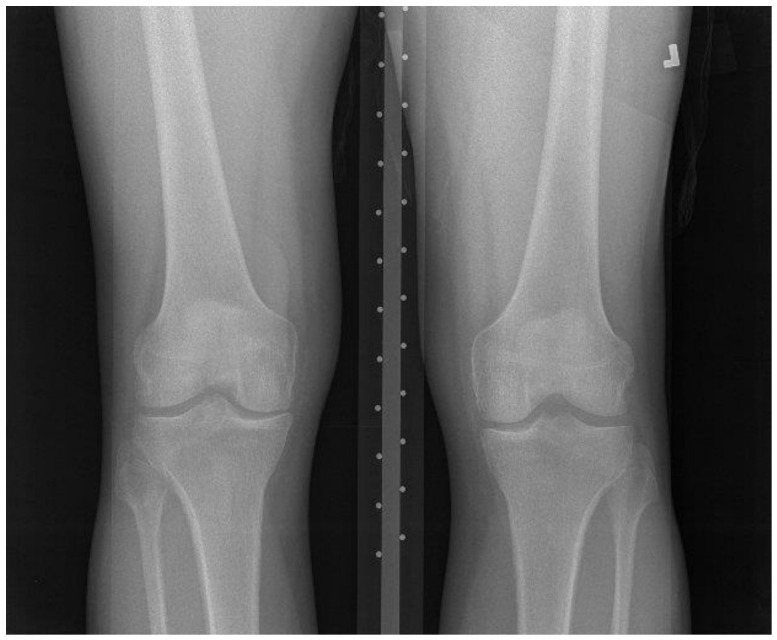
The knee X-ray image of a 65-year-old participant involved in volleyball across all four periods.
